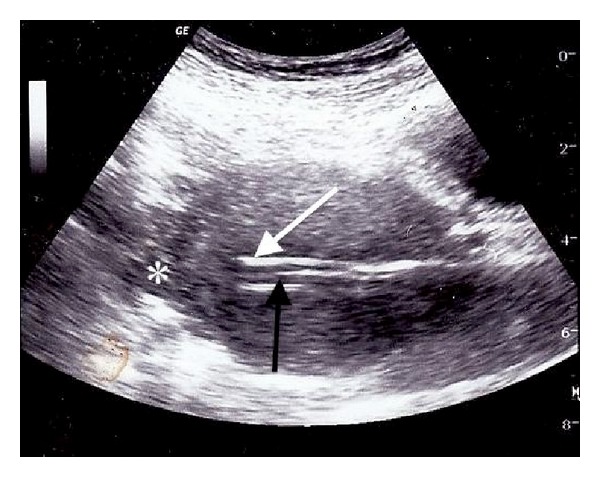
Under ultrasound guidance, the curette (white Arrow) was advanced over the stylet (black Arrow) into the uterine cavity (fundus star).
